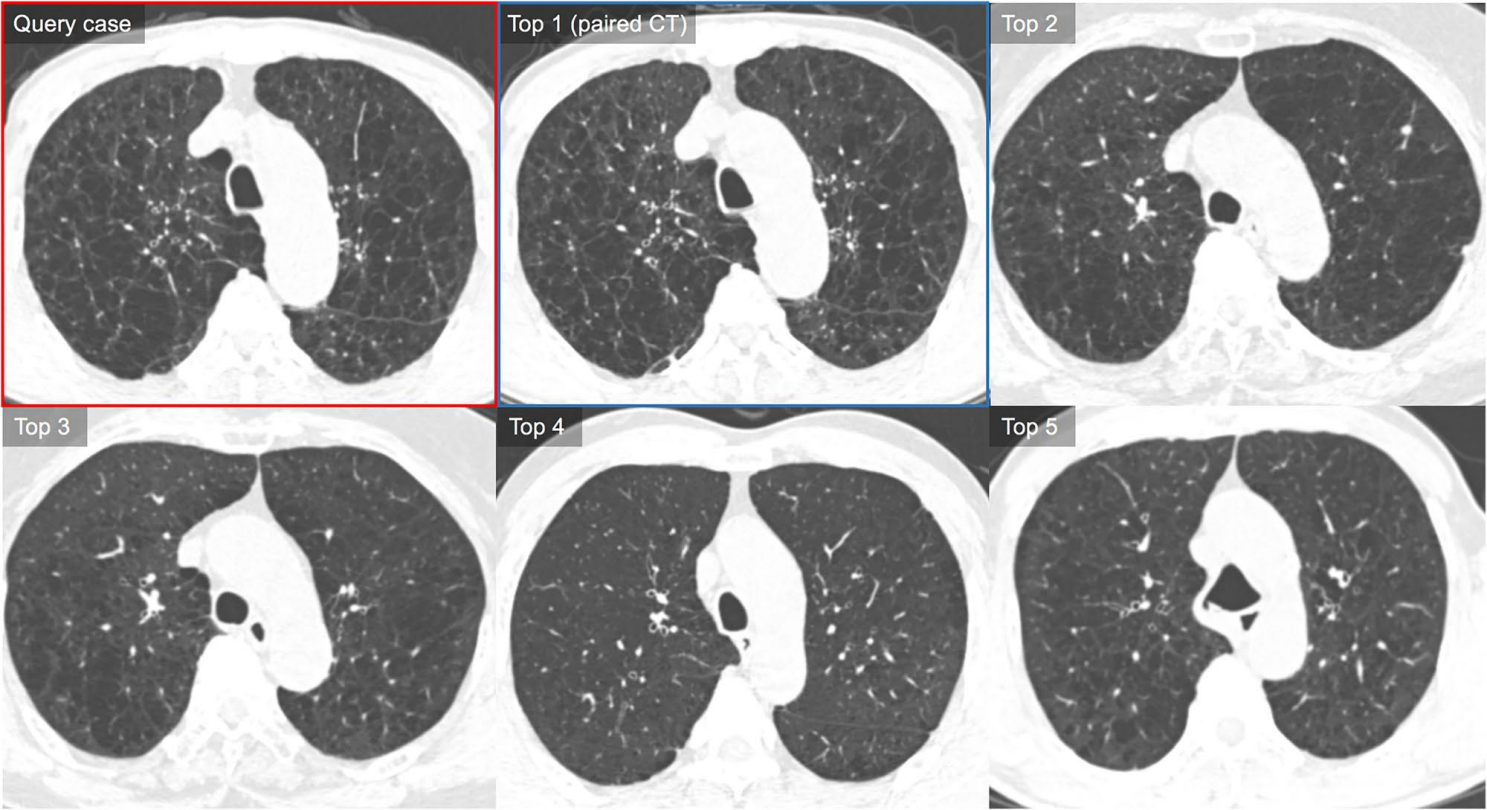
Representative case of the use of the content-based image retrieval (CBIR) system for chronic obstructive pulmonary disease. The query CT shows confluent centrilobular emphysema in both lungs with mild bronchial wall thickening (LAA = 23.1, D-slope = 4.3, PVV = 0.70, and Pi10 = 4.0). CBIR system retrieves five similar CTs based on the measured similarity in descending order. The query case (follow-up CT) is outlined with a red box and its paired case (a pair of baseline CT without interval change compared with the query case) among the retrievals is outlined with a blue box. Our CBIR system retrieved the baseline pair of query CTs from the database as the top 1 retrieval. CT images of top 2 to top 5 retrievals also show quite similar CT features showing mainly confluent or moderate centrilobular emphysema. In the visual similarity assessment, two chest radiologists rated the similarity scores of the top 1–5 as 5, 4, 4, 4, and 3, respectively, and the other chest radiologist as 5, 4, 5, 4, and 3.
